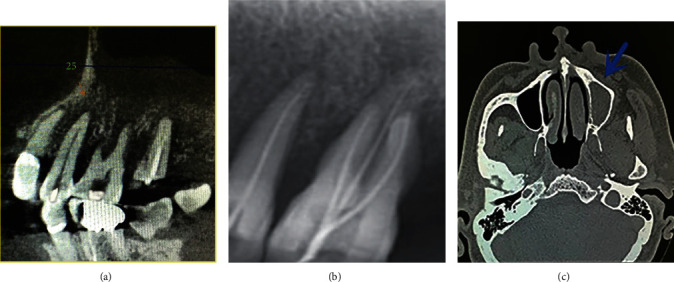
CBCT images showed multiple low-attenuating entities apical to premolars and the first molar. An extensive soft tissue mass was identified in the left maxillary sinus which extended through the medial maxillary sinus wall (a). Dental X-ray image showed appropriate root canal therapy (b). Transverse CT image showed maxillary sinus full of substantial objects which destroyed multiple walls (c).
